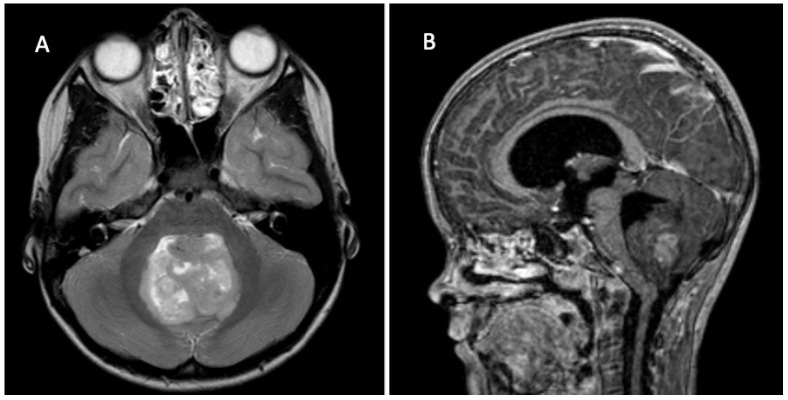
7 year old girl with signs of raised intracranial pressure and ataxia. (A) Axial T2 MRI shows midline tumor in the posterior fossa with IVth ventricle compression. (B) Sagittal contrasted T1 MRI with partially enhancing tumor and associated hydrocephalus. Diagnosis: Medulloblastoma, non-WNT/non-SHH (WHO grade 4).
